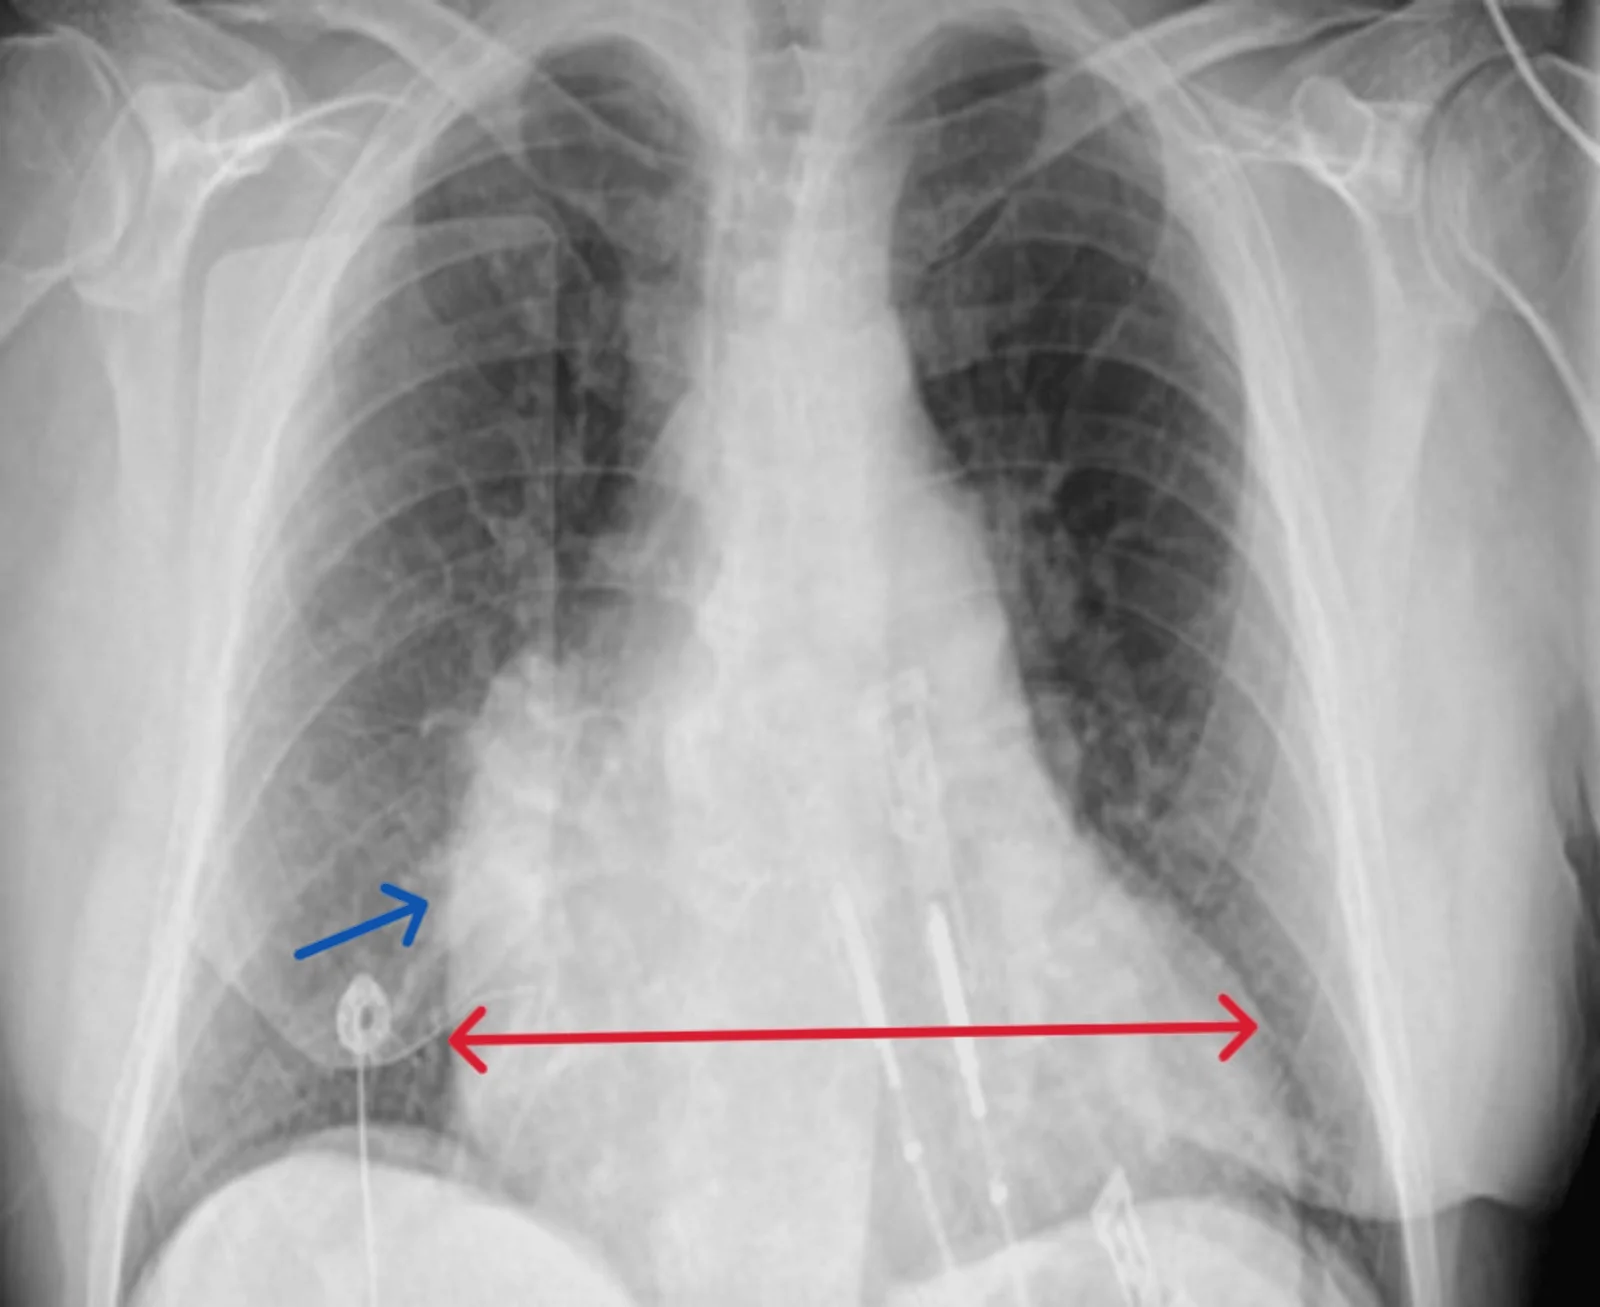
Chest X-ray showing cardiomegaly (red arrow) and enlarged right atrium (blue arrow)
